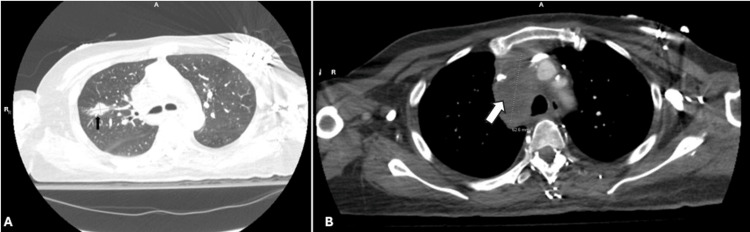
Chest CT findings. (A) An irregular nodule (black arrow) in the right upper lobe measuring 2.2 cm at its greatest dimension. (B) A hypodense mass (white arrow) in the superior mediastinum measuring approximately 6.3 cm at its greatest dimension.
